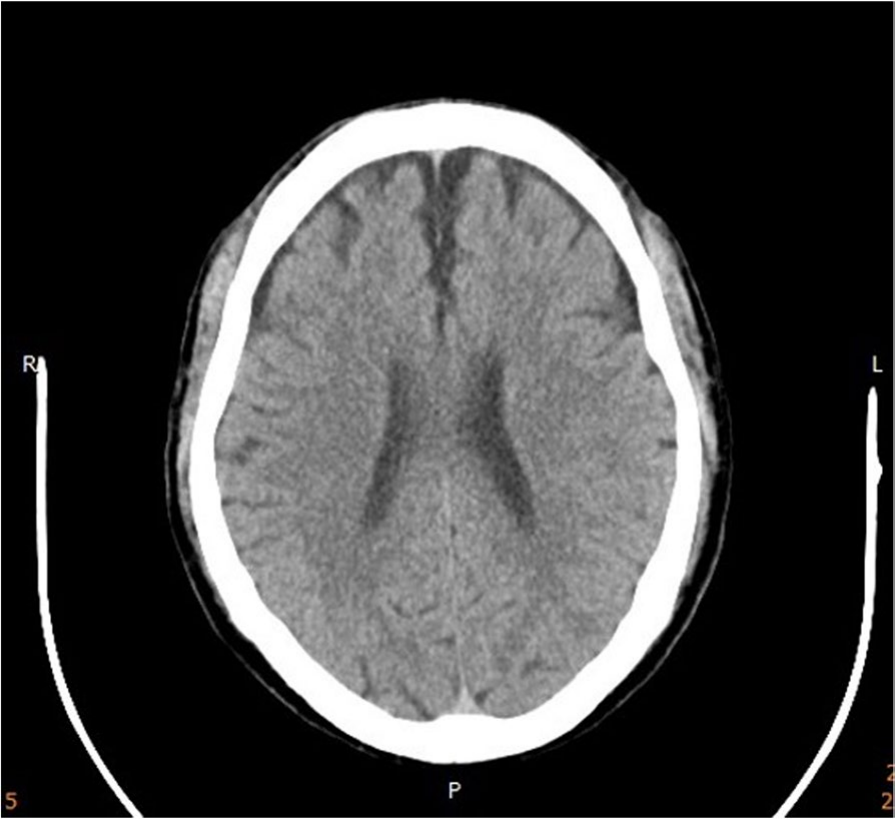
Post-seizure brain CT: Brain CT did not reveal remarkable bleeding or space occupying lesions
